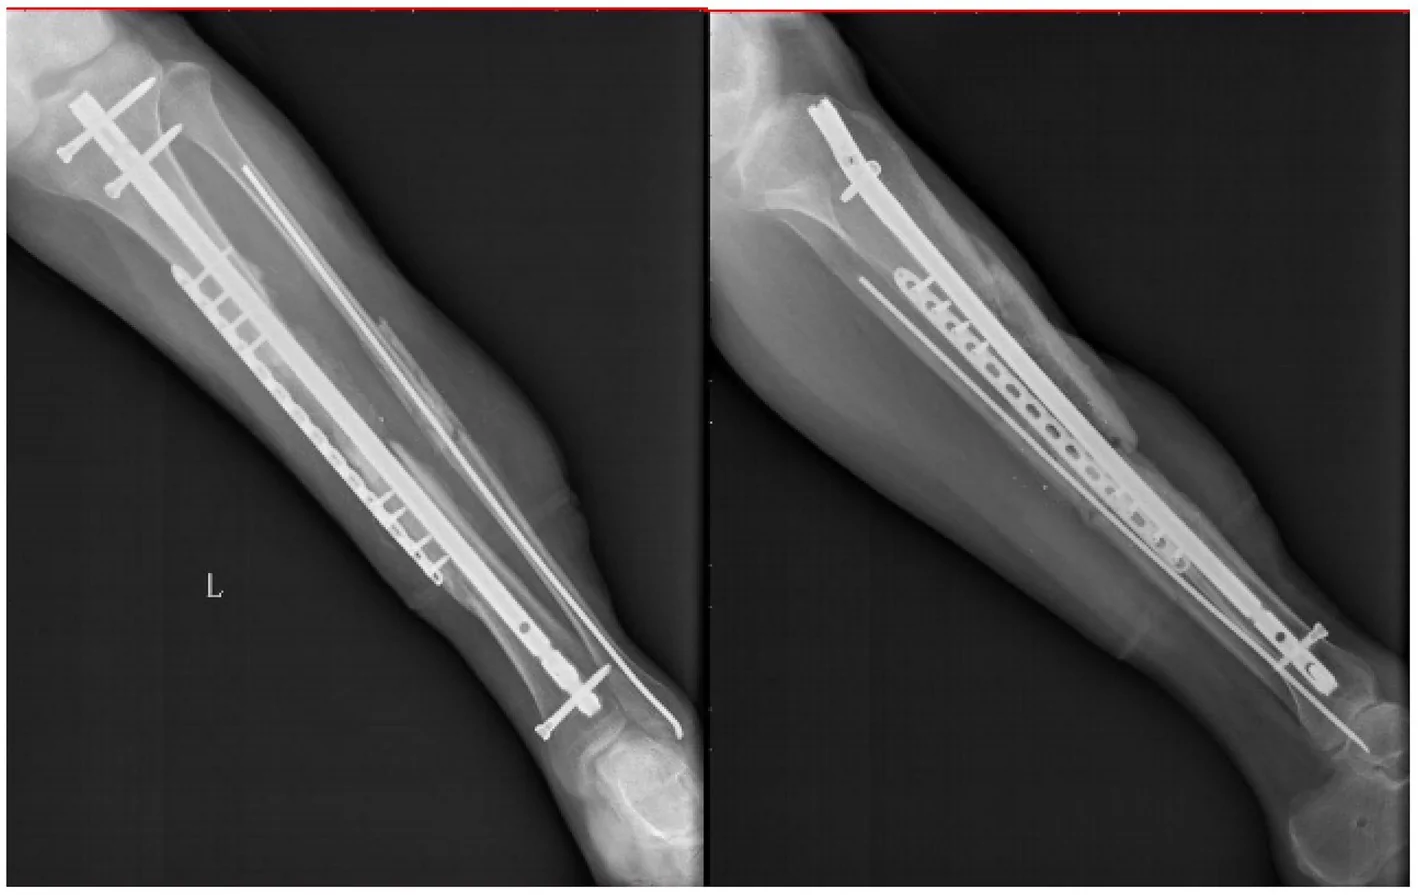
The X-ray findings one month after the bone grafting procedure.
